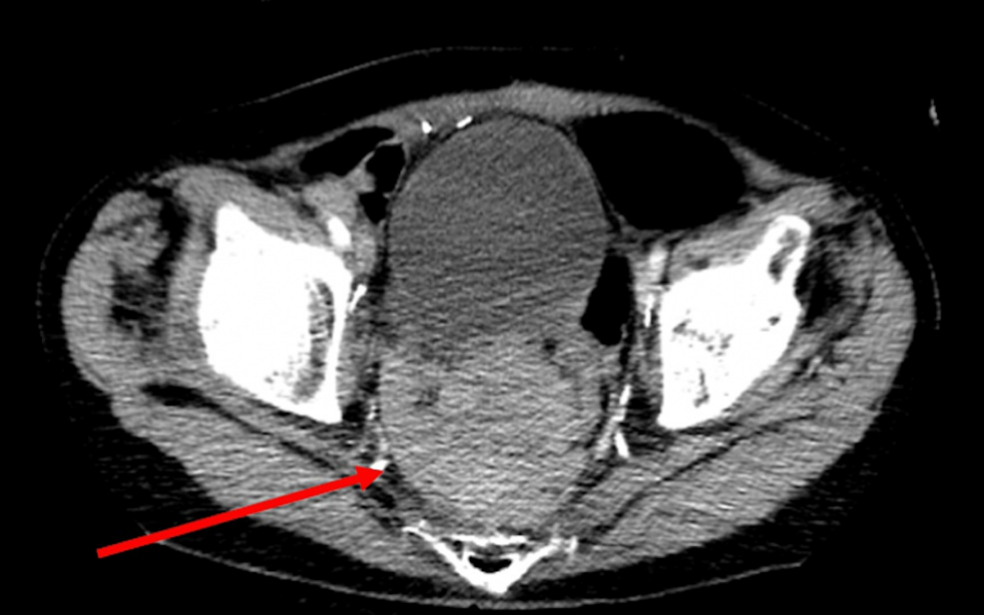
Contrast computed tomography (CT) of the pelvis showing a rectal mass Transverse view of the pelvis showing wall thickening and an enhancing mass lesion of the rectosigmoid colon obliterating tissue planes in the low pelvis and encasing the rectum measuring about 8 cm (red arrow).
